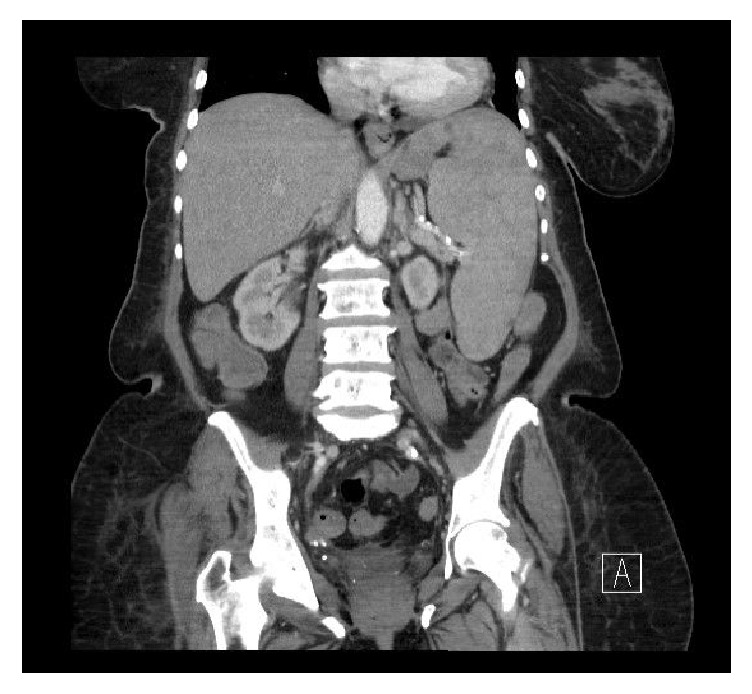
Abdominal and pelvic CT scan. Marked hepatomegaly and splenomegaly are identified. Spleen demonstrates numerous areas of heterogeneous hypoenhancement.
